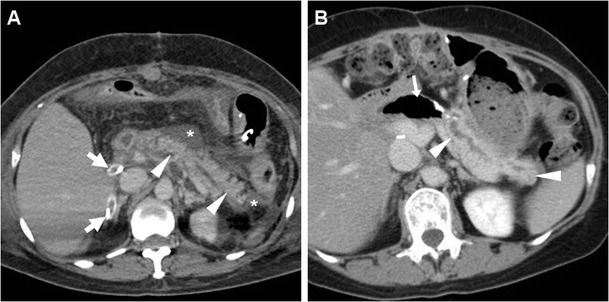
Delayed postoperative acute pancreatitis in the same 59-year-old woman as in Fig. 6, after early PJS dehiscence and surgical reintervention. Nearly a month after PD, CT (a) showed fluid (*) collecting around the medium-sized and normally enhancing RP, which ultimately resolved at follow-up (b) with residual MPD dilatation. Note drainage tubes (*thick arrows*), air-containing MJL (*arrow* in b)
